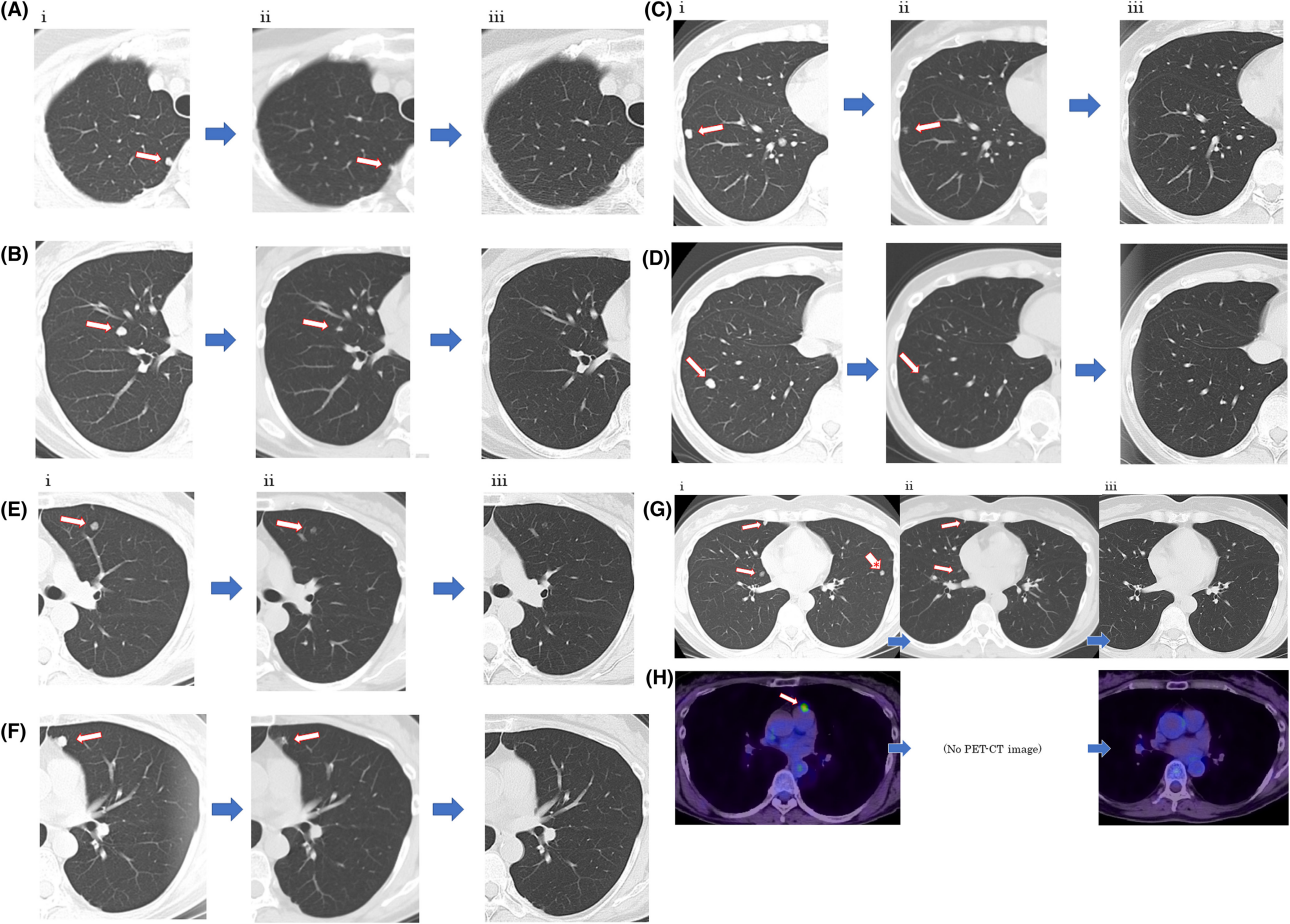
CT images of the lung and PET‐CT images of a para‐aortic lymph node. (A–G) Series i: CT images before AFTV treatment. Nine metastases (arrows) of uterine cancer are observed. (H, i) PET‐CT image indicating a small hotspot at the para‐aortic lymph node (arrow). (A–G) Series ii: Images after initial AFTV monotherapy. Three intradermal AFTV injections are administered 2‐week interval between each dose. The metastasis indicated with a red‐asterisk arrowhead (G, i) disappeared in (G, ii). No PET/CT images are available at H or in Series ii. (A–H) Series iii: Images after a one‐time injection of chemotherapy with carboplatin and docetaxel plus two additional injections with AFTV. A complete response is observed.
